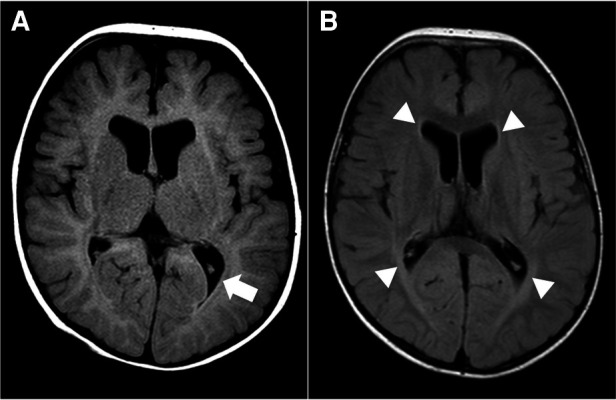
Brain magnetic resonance image (MRI) at 3 months (A) and 25 months (B) of age. (A) Axial T2-fluid attenuated inversion recovery (FLAIR) image of the brain demonstrates periventricular leukomalacia (arrow) with mild ventriculomegaly of the lateral ventricle. (B) Axial T2-FLAIR image of the brain shows abnormal high-signal intensity in the white matter, especially in the post-periventricular region and around the frontal horn (arrowhead). There is no interval change in ventriculomegaly of the lateral ventricle.
